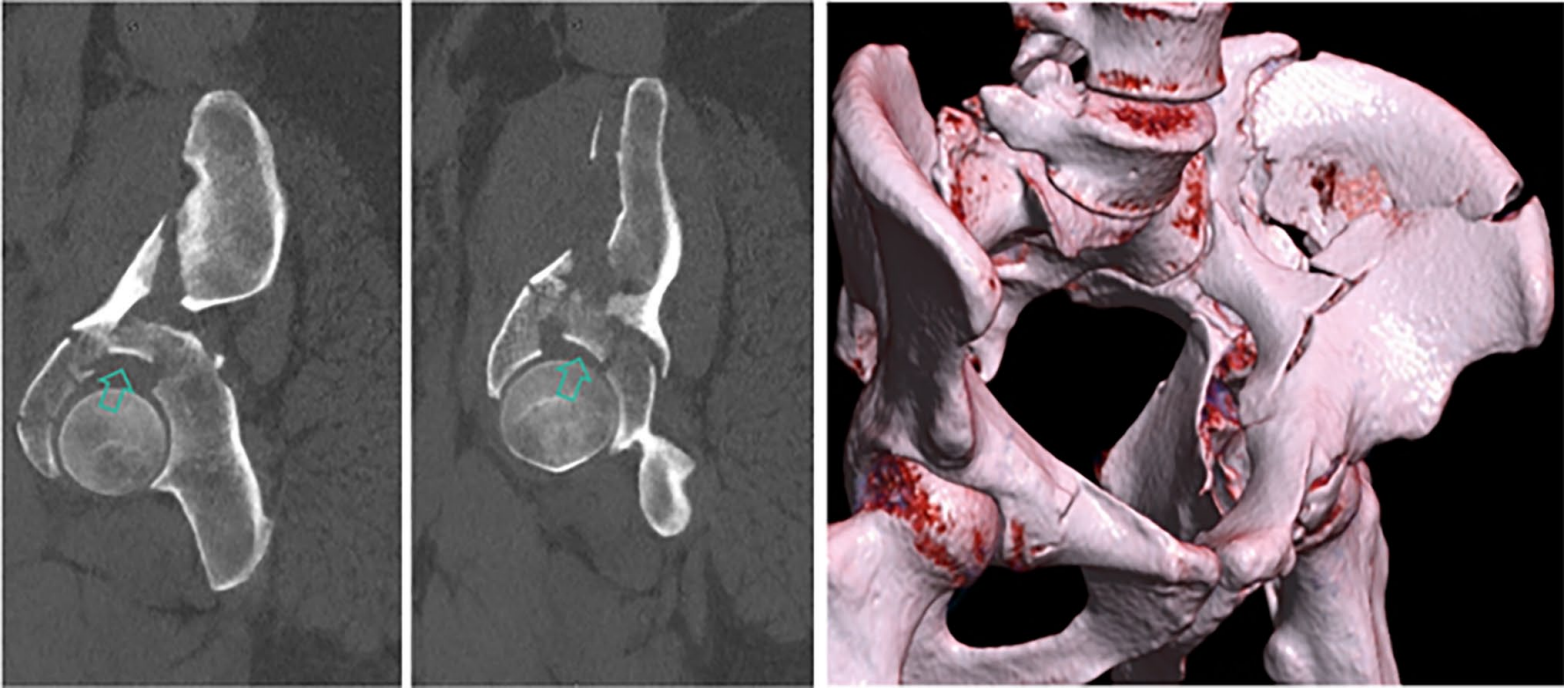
A 28-year-old man with severe obesity (BMI 367 kg/m^2^) fall from two meters’ height at work with direct trauma to the left side. The X-ray and the CT scan show a both column acetabular fracture with a large osteochondral fragment of the displaced acetabular roof (Fig. 1). Clinically there was a sciatic nerve injury associated. The large osteochondral fragment of the displaced acetabular roof is shown
